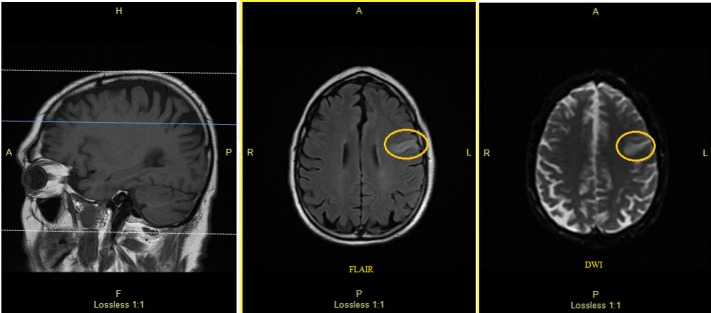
Magnetic resonance imaging (MRI) of head without contrast at presentation showing acute left middle cerebral artery territory infarction without midline shift or hemorrhagic conversion. Area of stroke marked by circles.
